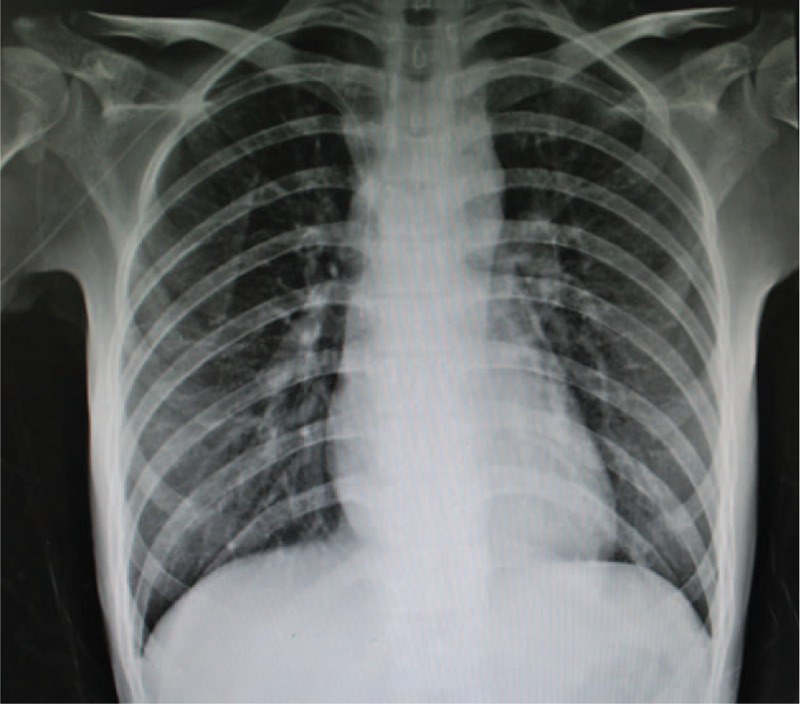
Follow-up x-ray after the first cycle of IA induction chemotherapy.
